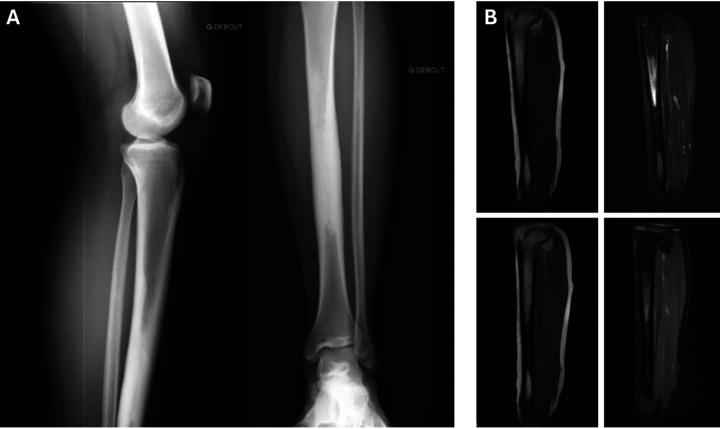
X-ray (A) and MRI (B) of the left tibia showing intramedullary osteosclerosis associated with peripheral edema.
